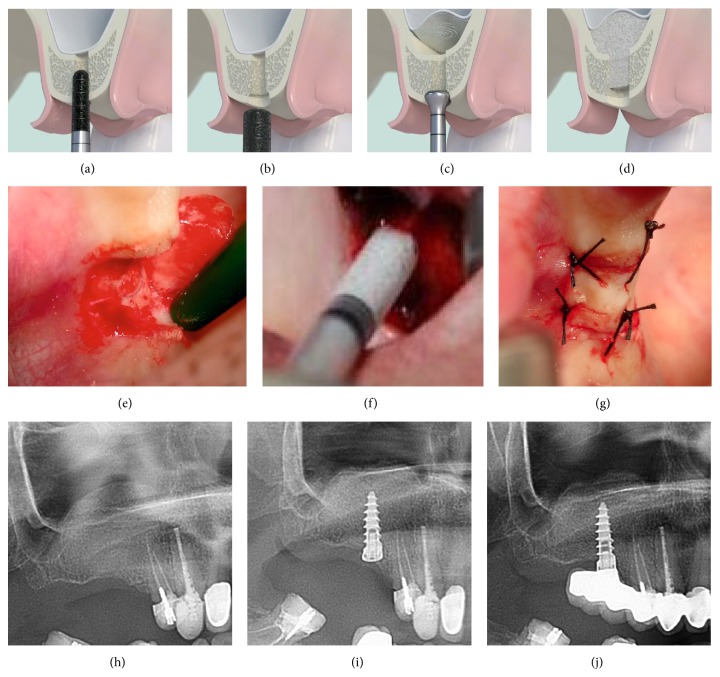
Overview tHUCSL-INTRALIFT procedure with Piezotome II/SOLO: (a) after reverting a minimal invasive crestal flap the sinus floor is opened transcrestal with the diamond coated Piezotome-tip TKW2, (b) a sealing receptacle is prepared with the diamond-coated Piezotome-tip TKW4, (c) the Piezotome-tip TKW5 is tight-fit inserted into the receptacle to prevent liquid-back-flow to the oral cavity and by this subantral loss of hydrodynamic pressure. The Piezotome then is activated and the periosteum of the Schneiderian membrane cleanly detached from the bony antrum floor utilizing the cavitation effect. (d) Insertion of 2 ccm biomaterial into the subperiosteal scaffold, (e) clinical case of a typical tHUCSL-INTRALIFT-procedure, (f) subantral application of 2 ccm biomaterial, (g) wound closure, (h) postsurgical X-ray control of the sinus lift site, (i) implant insertion after average 8.7 months, and (j) final prosthetic treatment.
